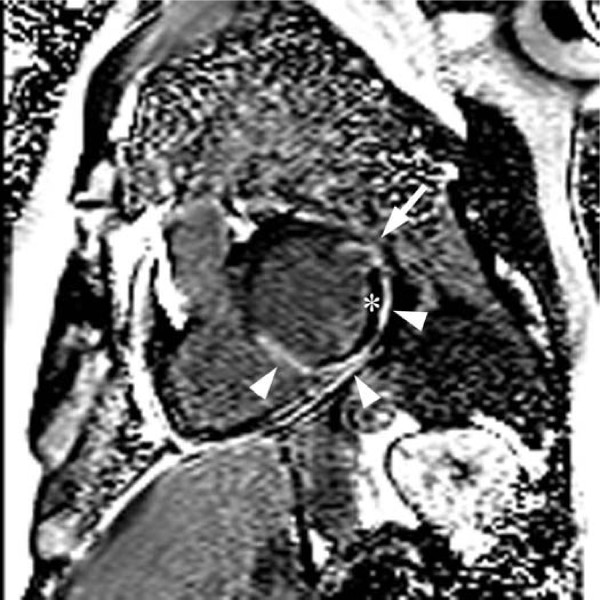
Short axis delayed enhancement inversion recovery MR image with phase correction after 55 minutes (SSFP-GRE) post intravenous gadolinium injection shows the large inferolateral and inferoseptal acute myocardial infarction (arrowheads) with a persistent large area of microvascular obstruction (*). The impending rupture site in the lateral left ventricular wall shows delayed enhancement of the thin overlying cover of infracted myocardium (arrow).
